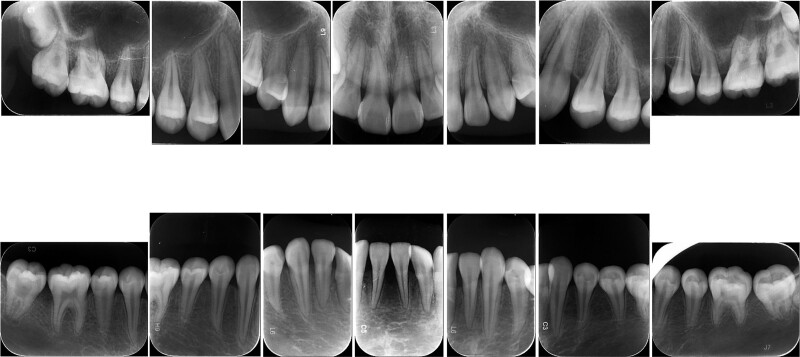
Periapical radiographs showing the formation of the bone-white line.
